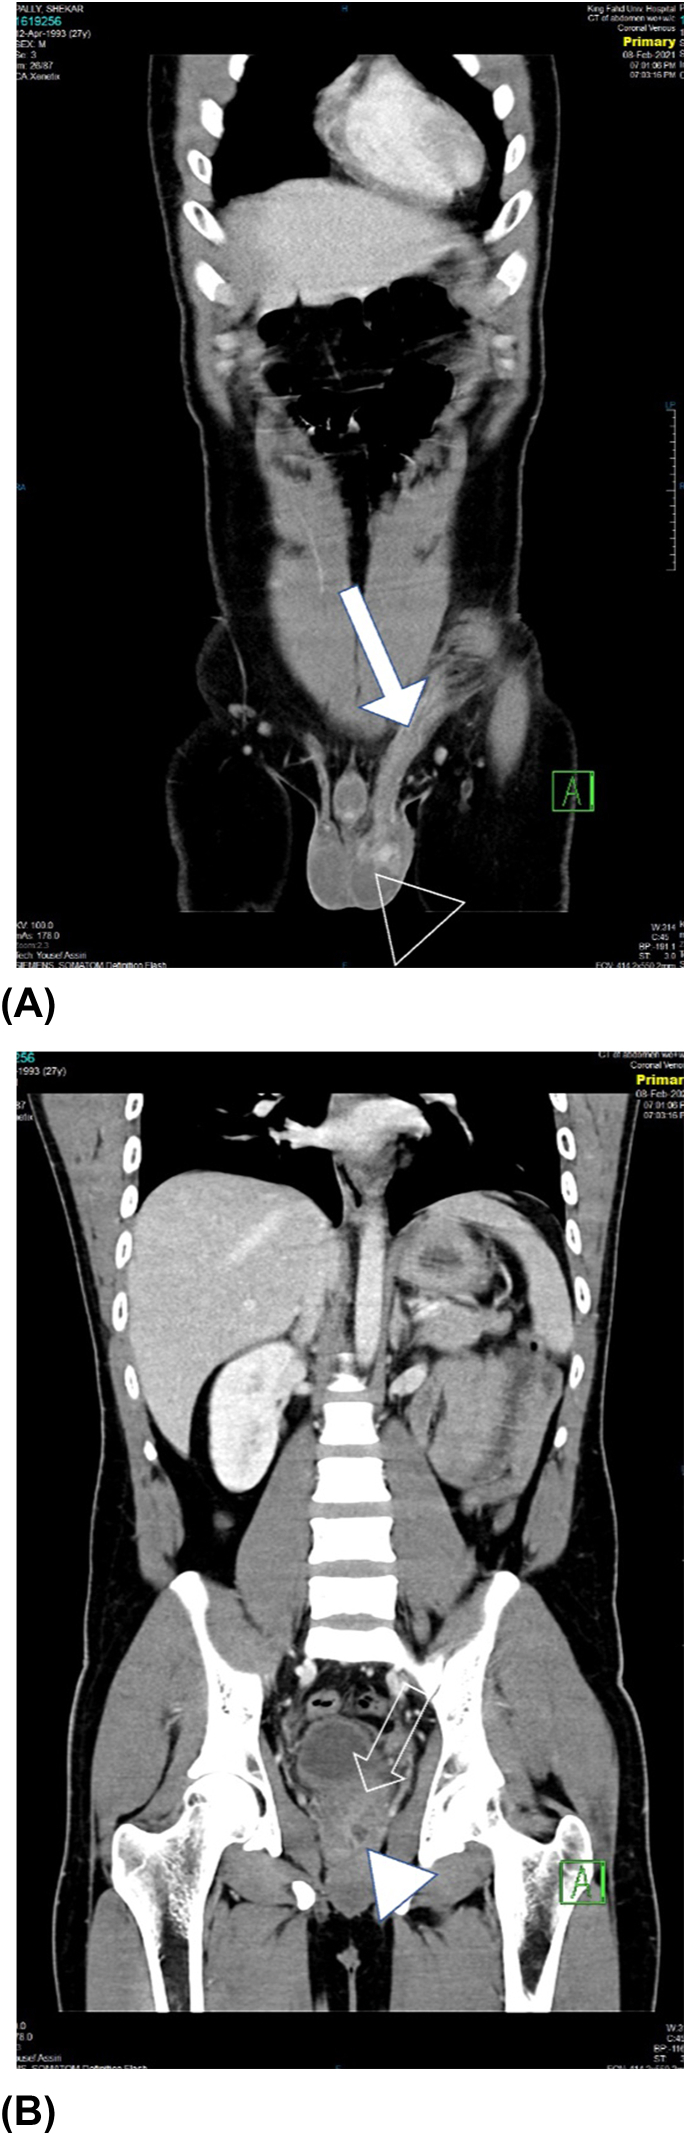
(A, B) Contrast enhanced CT scan coronal images showing hyperenhancement of the left epididymal head (empty arrow head) with vascular engorgement, swelling and significant thickening of the left spermatic cord (solid arrow). This inflammation is seen extending more proximally along the left inguinal canal. The left seminal vesicle (empty arrow) showing increase enhancement with a small rim enhancing collection (arrow head) in its most inferior aspect measuring 1.5*2.8 mm in maximum dimension.
